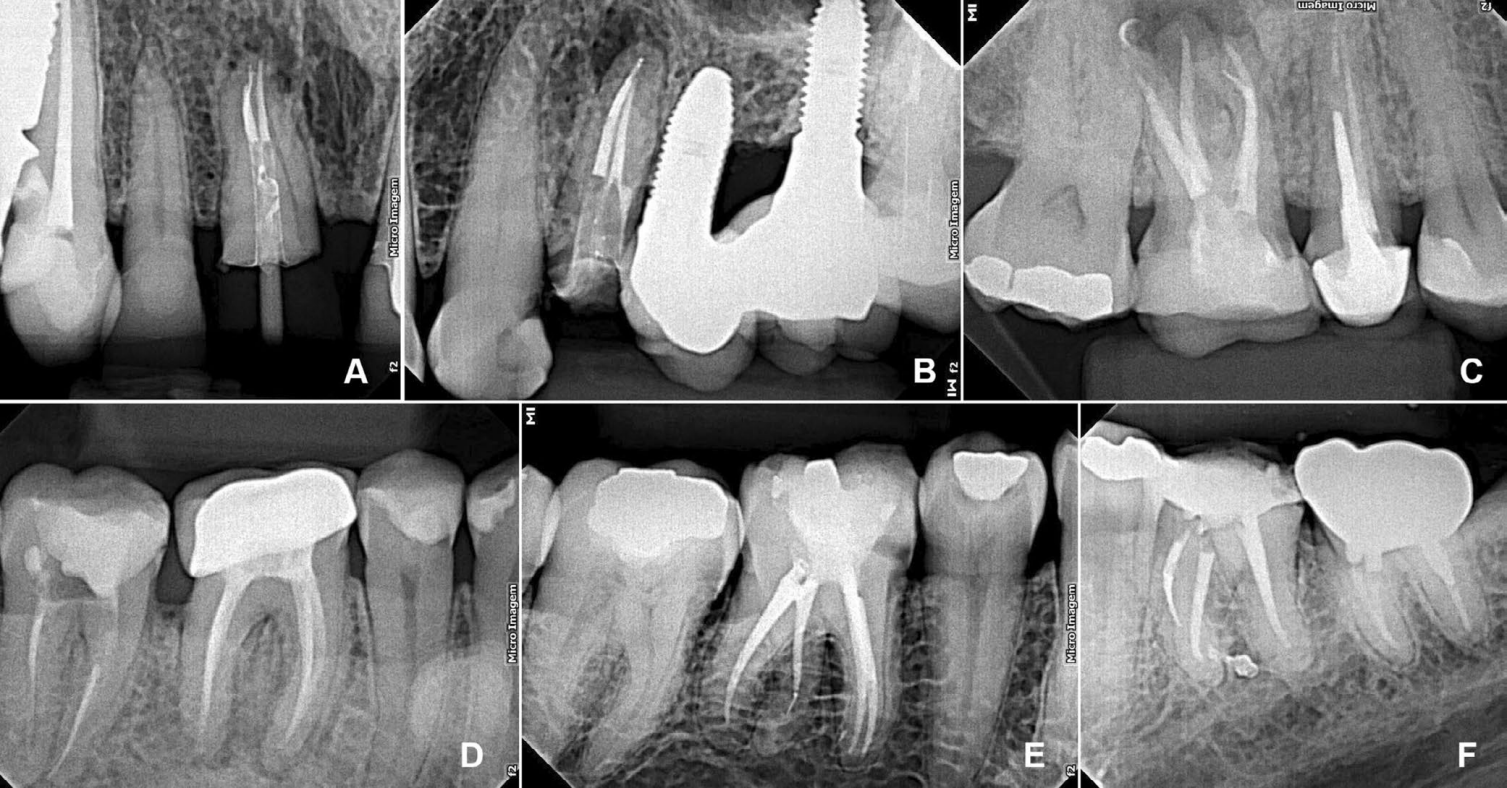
Radiographic images of the six fractures using R25 and X1 Blue instruments. (A) Fracture of an R25 instrument, used for the first time, in the apical third of the P canal in a maxillary central incisor. (B) Fractured R25 instrument in its second use, in the apical third of the P root canal of a maxillary premolar. (C) X1 Blue file, in its first use, fractured in the apical portion of the P root canal of a maxillary first molar. (D) Fractured X1 Blue in its first use in the apical third of the MB canal of a mandibular second molar. (E) Fractured R25 file, used three times, located in the apical third of the DL canal of a mandibular first molar. (F) Fractured R25 file during its first use, in the apical third of ML canal of a maxillary first molar.
